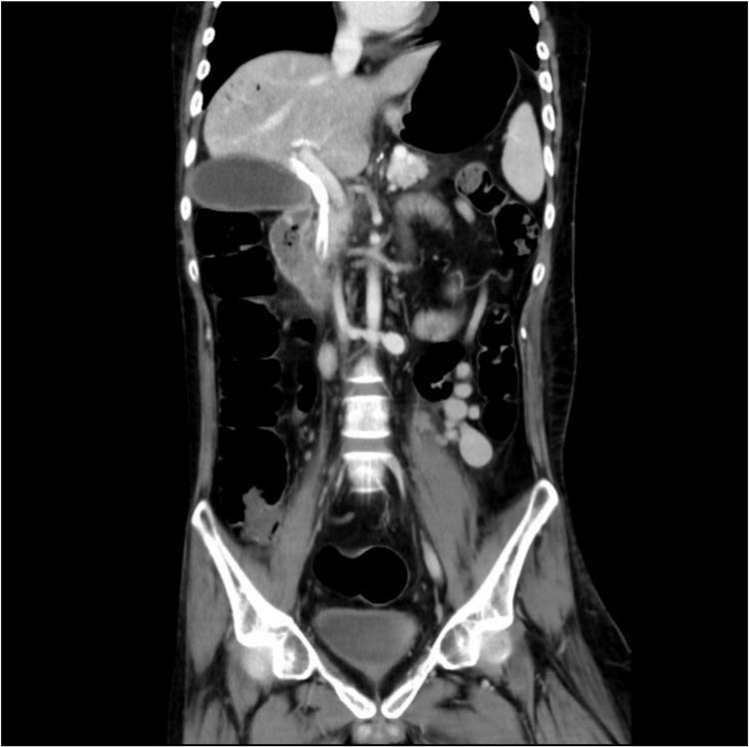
Just after ERCP, the abdominal CT showed retained contrast medium in bile duct.
